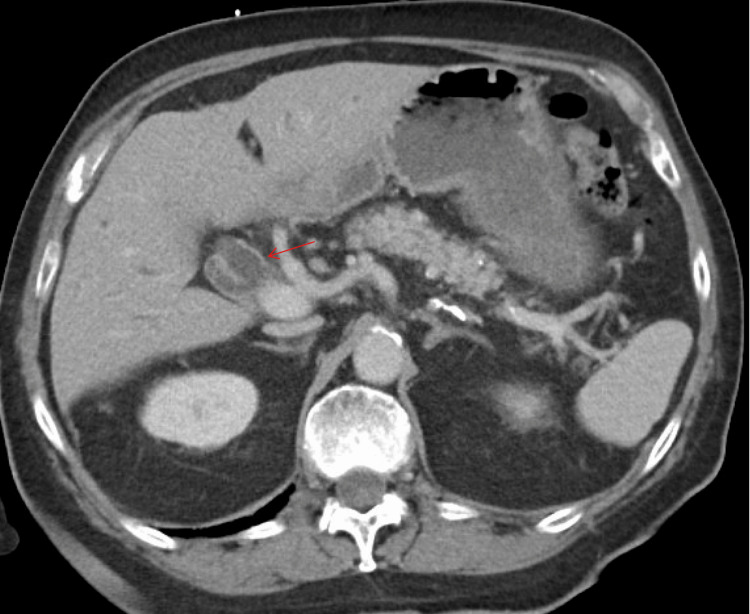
CT abdomen and pelvis, axial view in venous phase. The red arrow points at a moderately dilated common bile duct measuring 15 mm, with associated intrahepatic ductal dilation.
